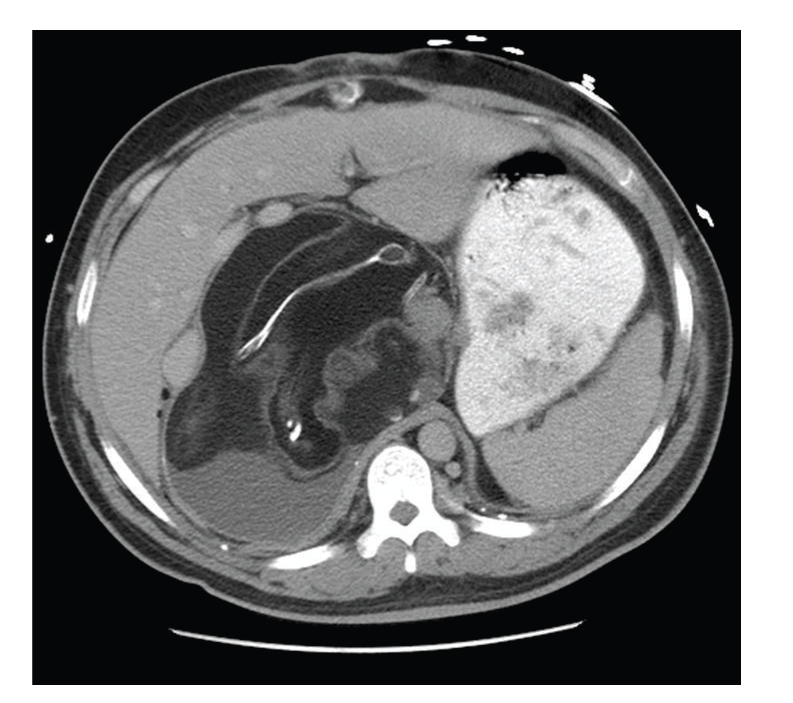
CT scan superior to the umbilicus shows a large, right-sided retroperitoneal mass with bone and soft tissue.
